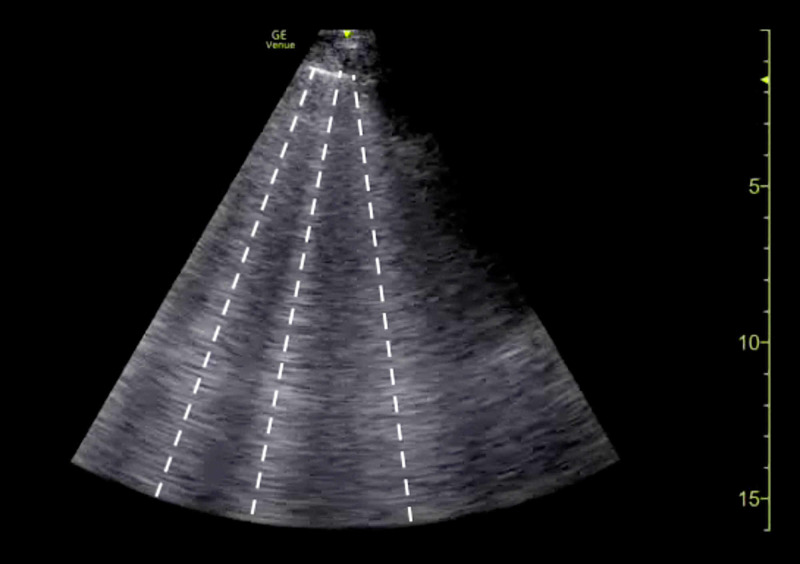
Point-of-care lung ultrasound demonstrating a B-line profile (white lines).
